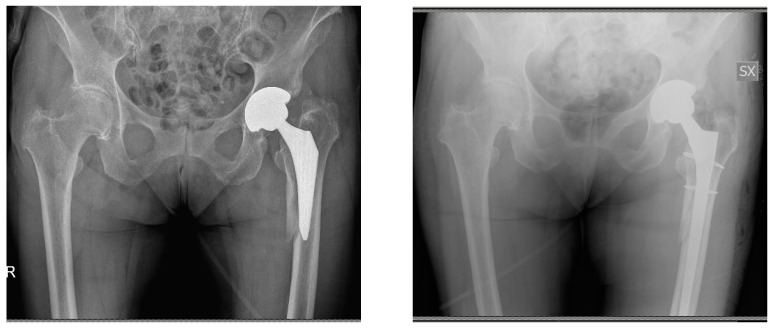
An 85-year-old female patient. Periprosthetic fracture of the GTS (Zimmer Biomet) stem two years after implantation (left). Postoperative x-ray after revision surgery with Wagner Revision stem (Zimmer Biomet) implant (right).
